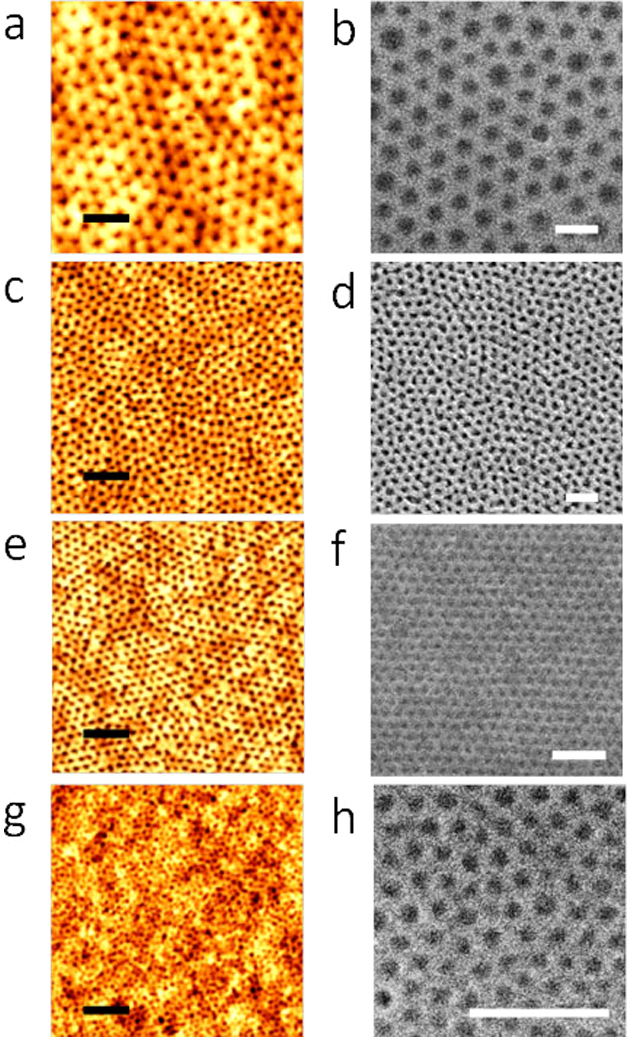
AFM and SEM images of the nanoporous template after ethanol ultrasonication for different periods of time (a), (b) 102 k–34 k, 20 min (c), (d) 42 k–11.5 k, 17 min (e), (f) 32 k–11 k, 15 min and (g), (h) 16 k–5 k, 10 min respectively. (a), (c), (e), (g) scale bar: 200 nm. (b), (d), (f), (h) scale bar: 100 nm.
